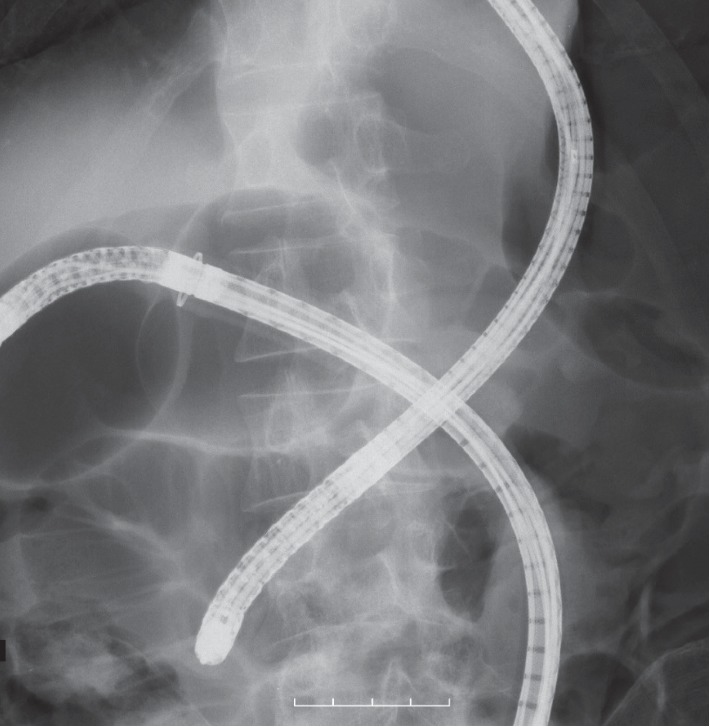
The X-ray image of the collaborative work with the gastrointestinal fiberscope and colon fiberscope. We abandoned the attempt to insert the PEG because the transverse colon is flopped over the stomach.
